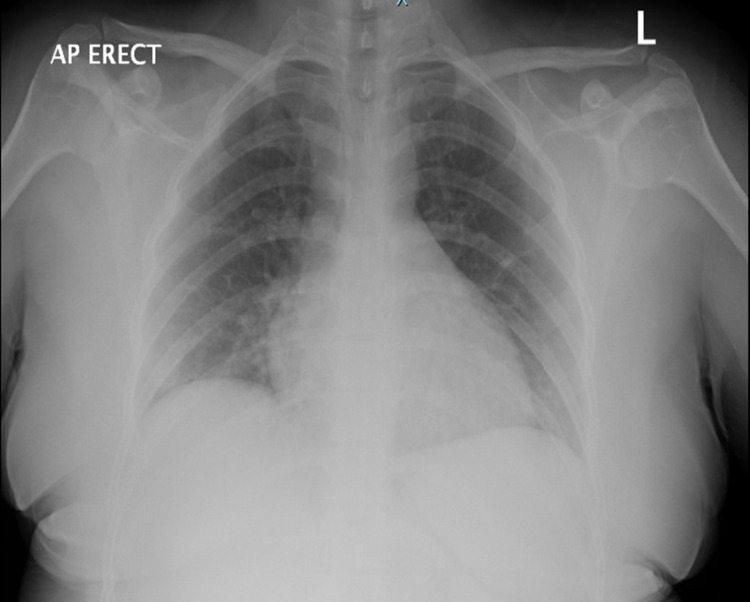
Chest x-ray is negative for ground-glass opacities that are classically present in COVID-19 pneumonia.
